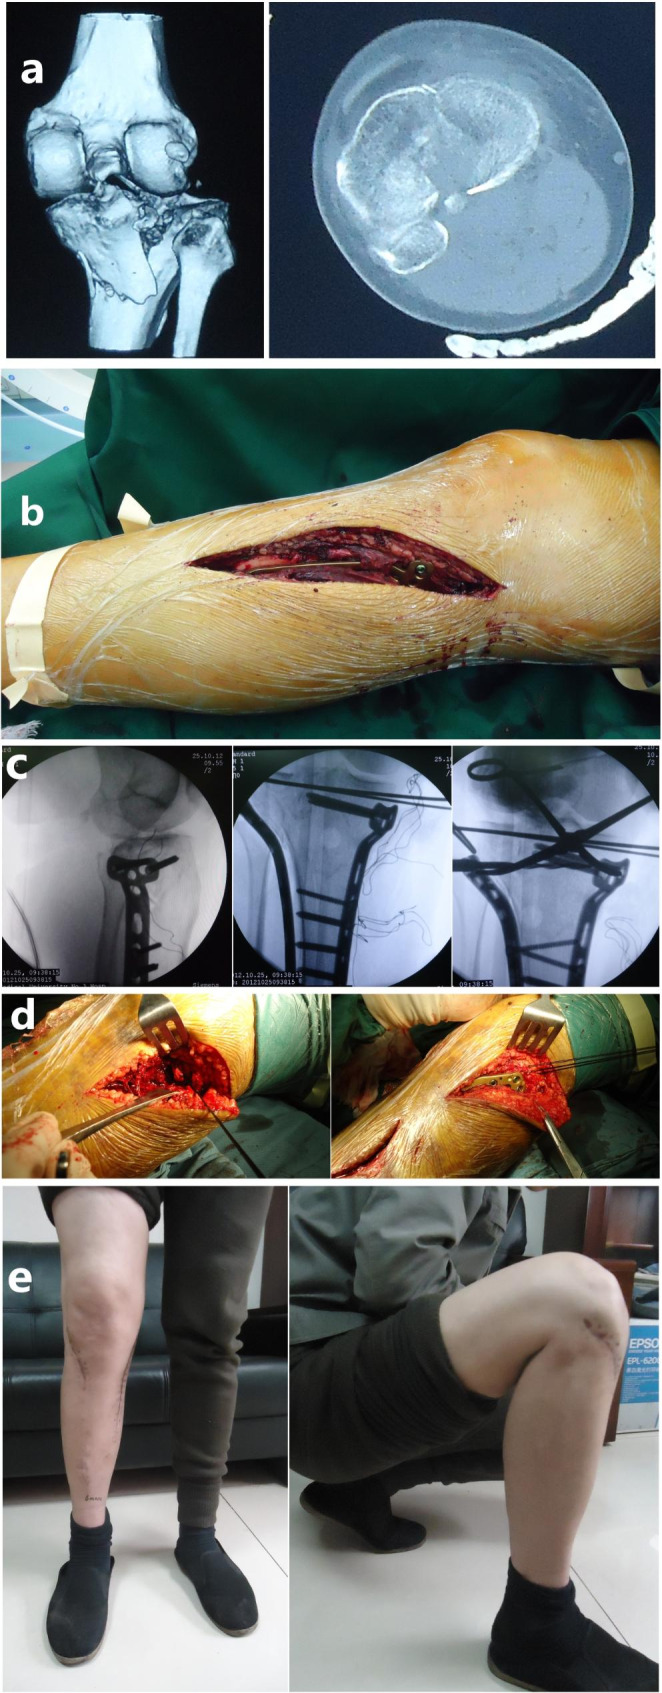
A typical case of a 39-year-old male with Schatzker IV tibial plateau fractures (flexion-valgus-internal rotation type). (a) The features of this type were shown in CT images. (b) Medial approach was needed for the reduction of MFA. (c-d) A posteromedial incision was made to expose the posteromedial fracture and MFA in a supine position. After the reduction, the MFA was fixed by screws and a plate that was placed upon the MFA. Then a lateral incision was made to expose MCZ located in posterolateral quadrant and to explore the displaced lateral meniscus. The displaced lateral meniscus and the lateral joint capsule were sutured together. Bone grafting combined with internal fixation was performed after the reduction of the lateral articular surface. (e) Postoperatively, the function of the knee was excellent
